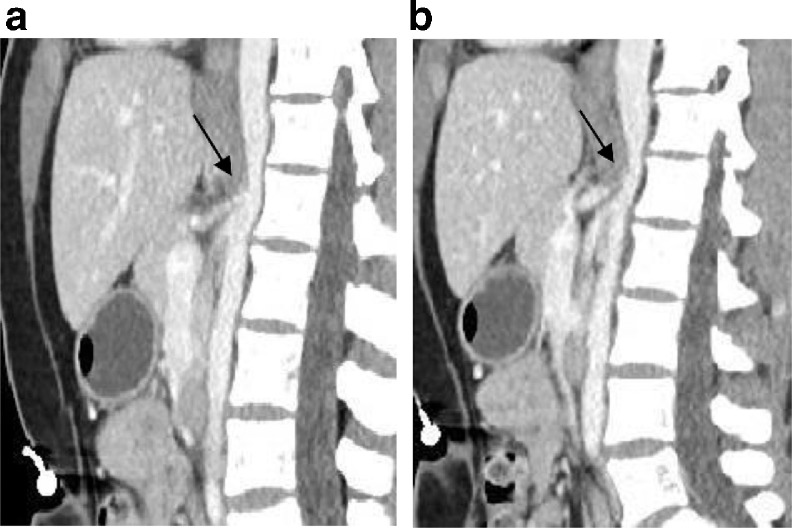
(A): Contrast-enhanced CT of the abdomen, parasagittal reconstruction, shows kinking of the proximal celiac artery, resulting in characteristic hooked appearance with post stenotic dilatation. Note the absence of atherosclerosis. (B): Contrast-enhanced CT of the abdomen, parasagittal reconstruction, shows post stenotic dilatation of the celiac artery.
